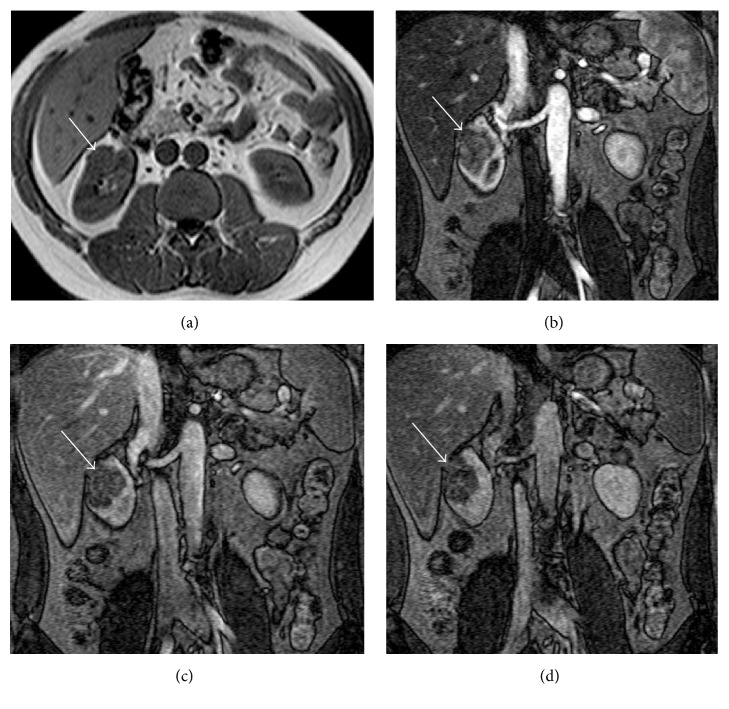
P-RCC. Axial GE T1-weighted image (a); coronal GE T1-weighted dynamic contrast-enhanced MR scans (b–d). Exophytic isointense nodule (arrow) in the right kidney with poor enhancement in multiphasic study.
